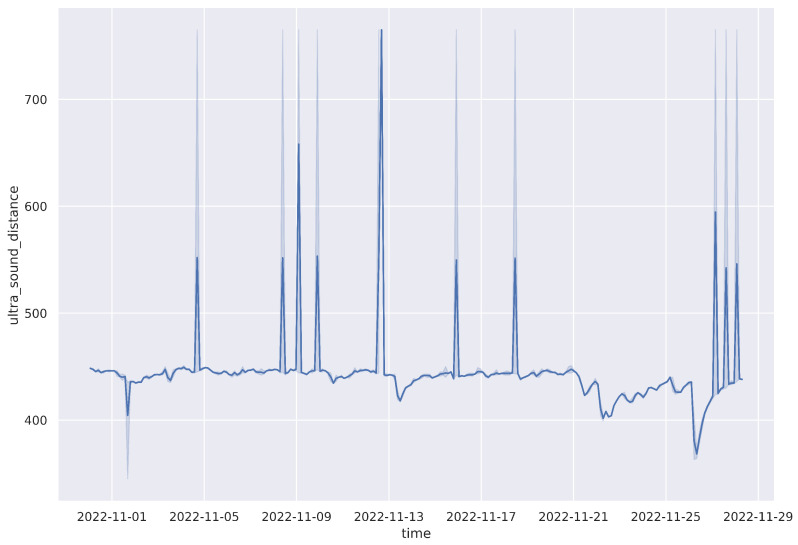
Ultrasound data plot for November 2022 at Kikuletwa bridge.
